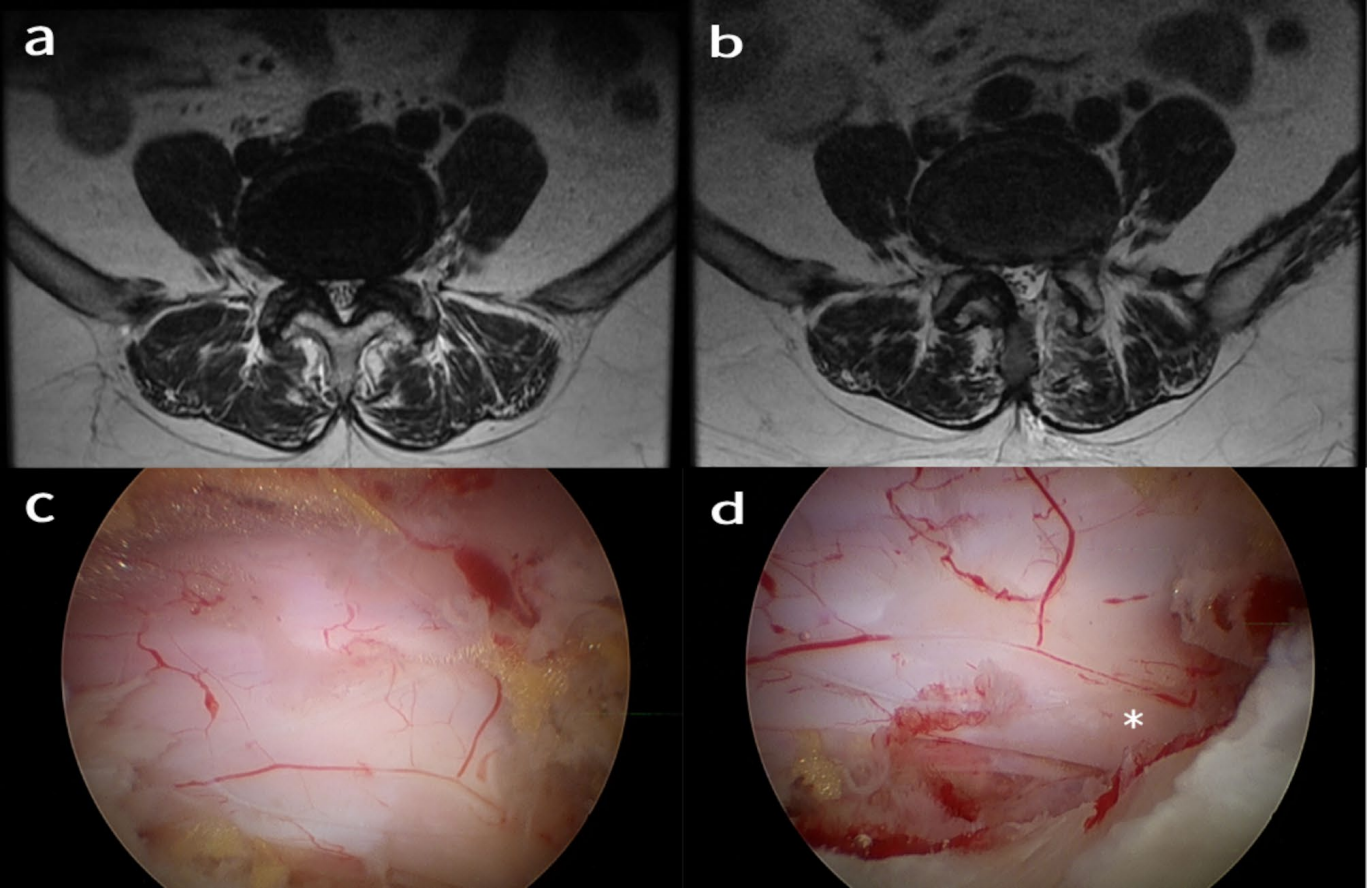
Full-endoscopic unilateral lumbar decompression via interlaminar approach in a 66-year-old female patient. (a) Preoperative MRI demonstrating central canal stenosis at the L4-5 level. (b) Postoperative MRI revealing complete decompression of the dural sac on the left side. (c, d) Intraoperative endoscopic views showing the decompressed dural sac and ipsilateral nerve root (asterisk)
